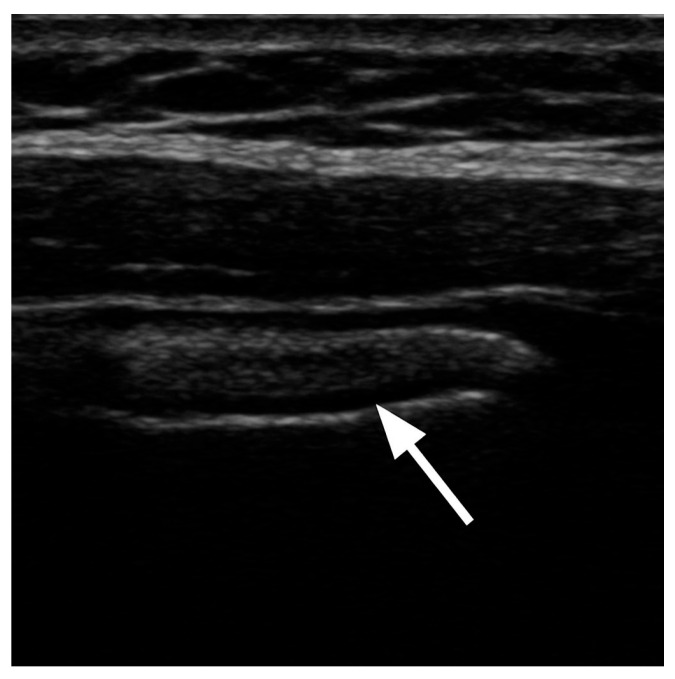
Ultrasound image of the fibularis tertius muscle in a lateral view, with the patient lying in a supine position. The muscle is marked with an arrow.
